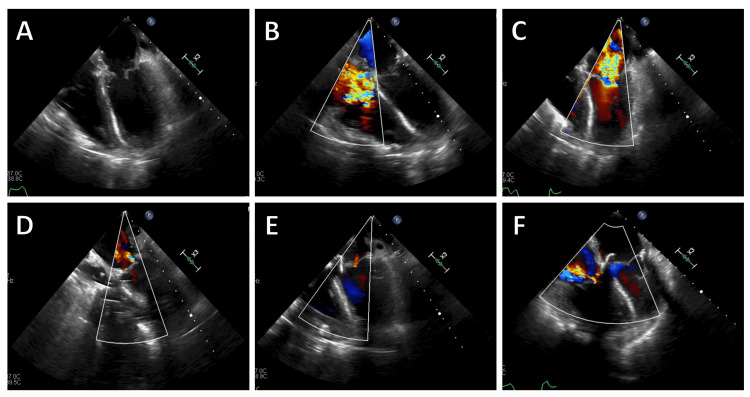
Intraoperative transesophageal echocardiography (TEE) images. (A) After removal of the myxoma, (B) severe tricuspid regurgitation, (C) severe mitral regurgitation, (D) persistent significant regurgitation after mitral valve repair, (E) result after mitral valve replacement, (F) residual less significant tricuspid regurgitation after tricuspid valve annuloplasty.
